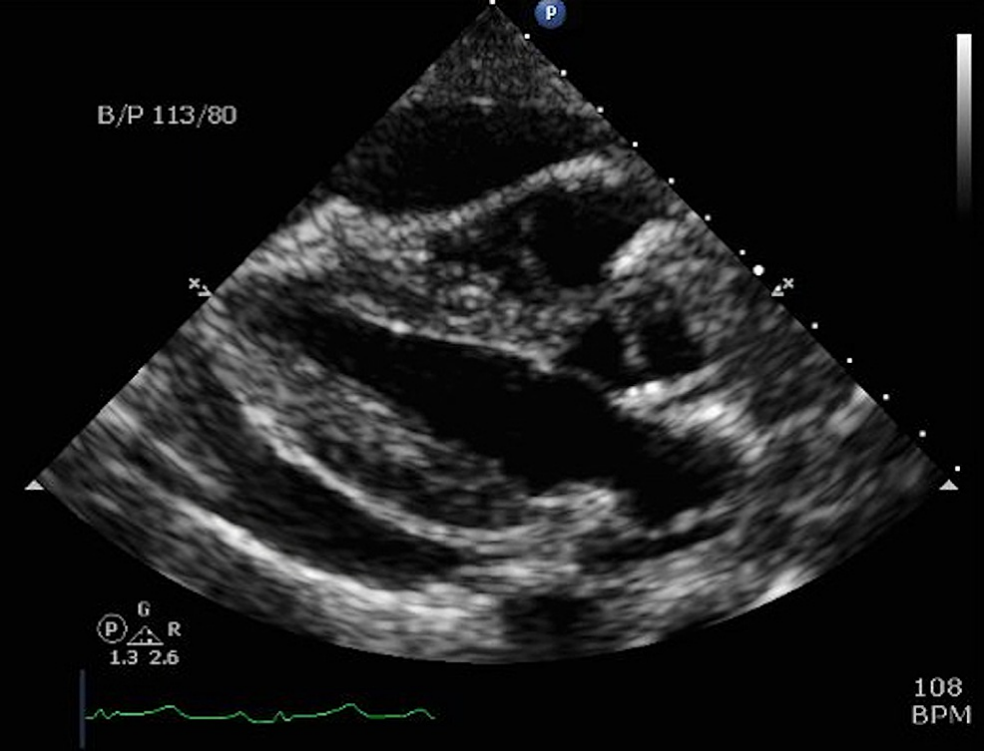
2D transthoracic echocardiography showing a large circumferential pericardial effusion in the parasternal long-axis view.
